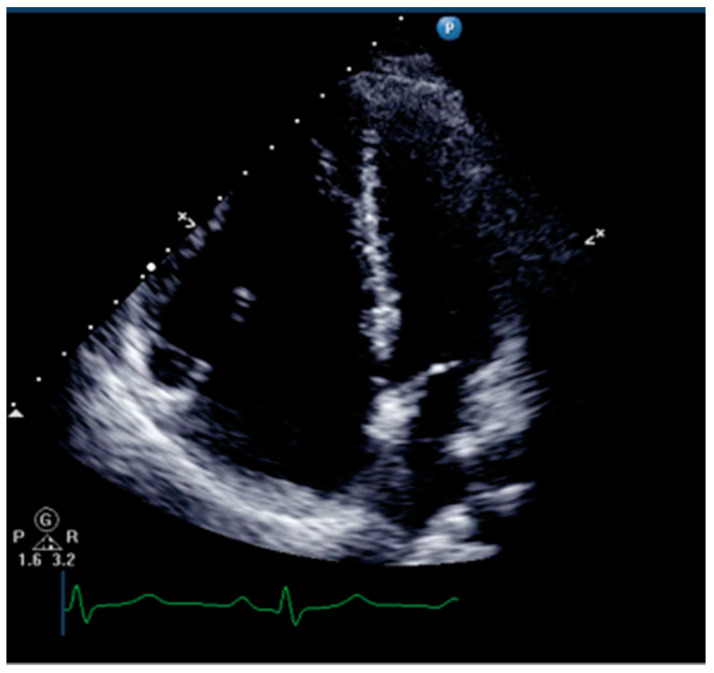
The ultrasound sign of right ventricular dilation.
